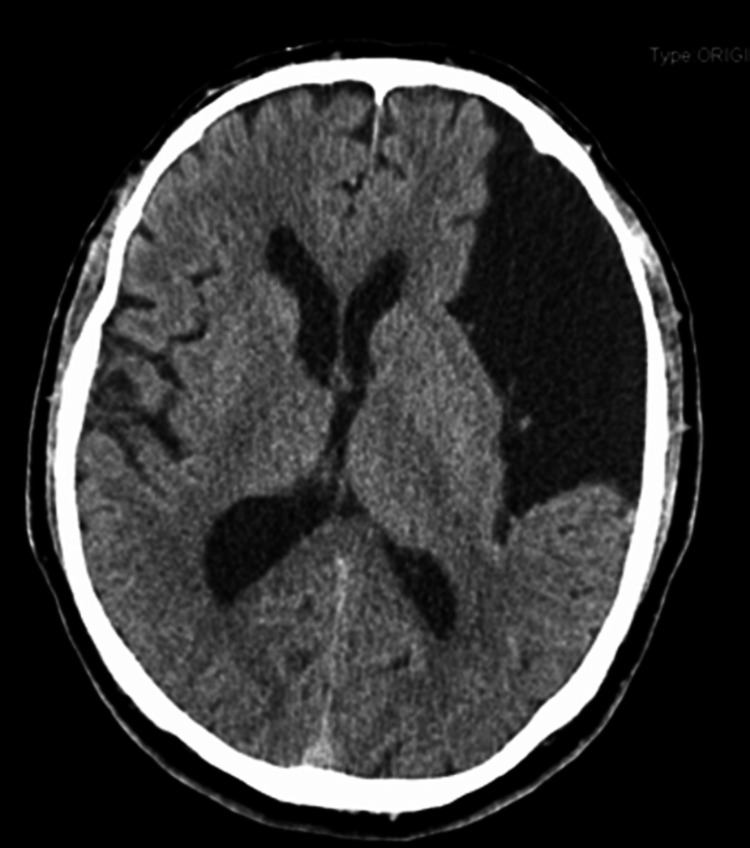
Brain computed tomography Brain computed tomography in axial view showing a large left fronto-parieto-temporal extra-axial fluid collection measuring 9.5 x 5.1 cm of maximum diameters creating a significant parenchymal and ventricular compression with subfalcine herniation (midline shift). It is also possible to see a 5 mm deviation of midline structures.
